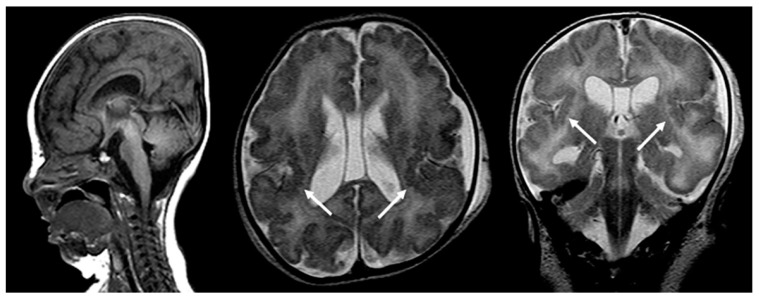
Sagittal T1-weighted, and axial and coronal T2-weighted MRIs of a neonate with confirmed Zellweger syndrome. Bilateral perisylvian polymicrogyria is noted (arrows), along with mild T2-hyperintense hypomyelination of the hemispheric white matter, mild ventriculomegaly, and frontal bossing.
